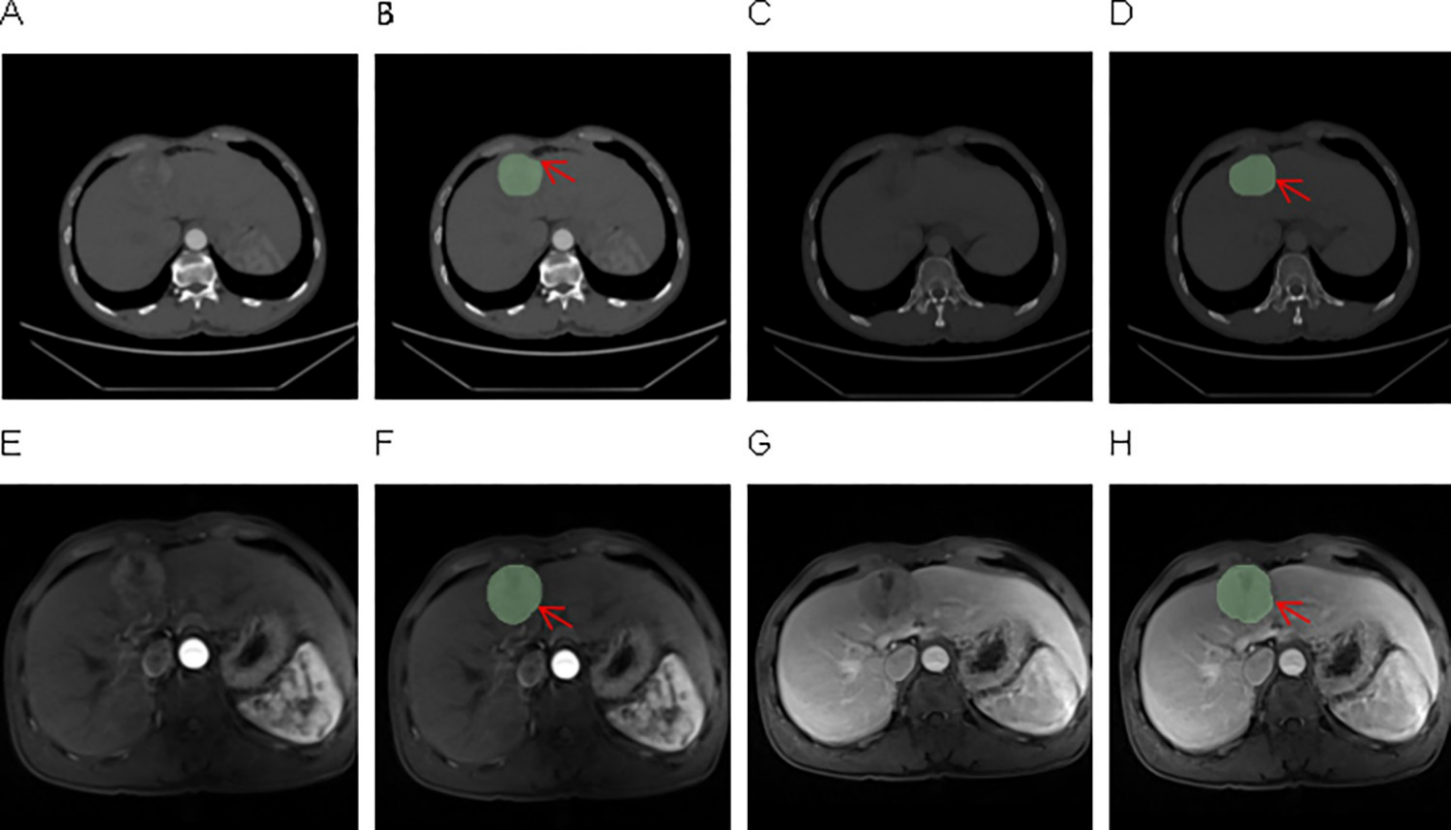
Extracted CT and MRI images and outlined ROI. (A) CT arterial phase images. (B) ROI sketched by CT in arterial phase. (C) CT venous phase images. (D) The ROI outlined in the venous phase of CT. (E) The arterial phase image of MRI. (F) ROI sketched by MRI in arterial phase. (G) The venous phase image of MRI. (H) The ROI outlined in the venous phase of MRI. ROI: The region of interest; CT: Computerized tomography; MRI: Nuclear magnetic resonance.
